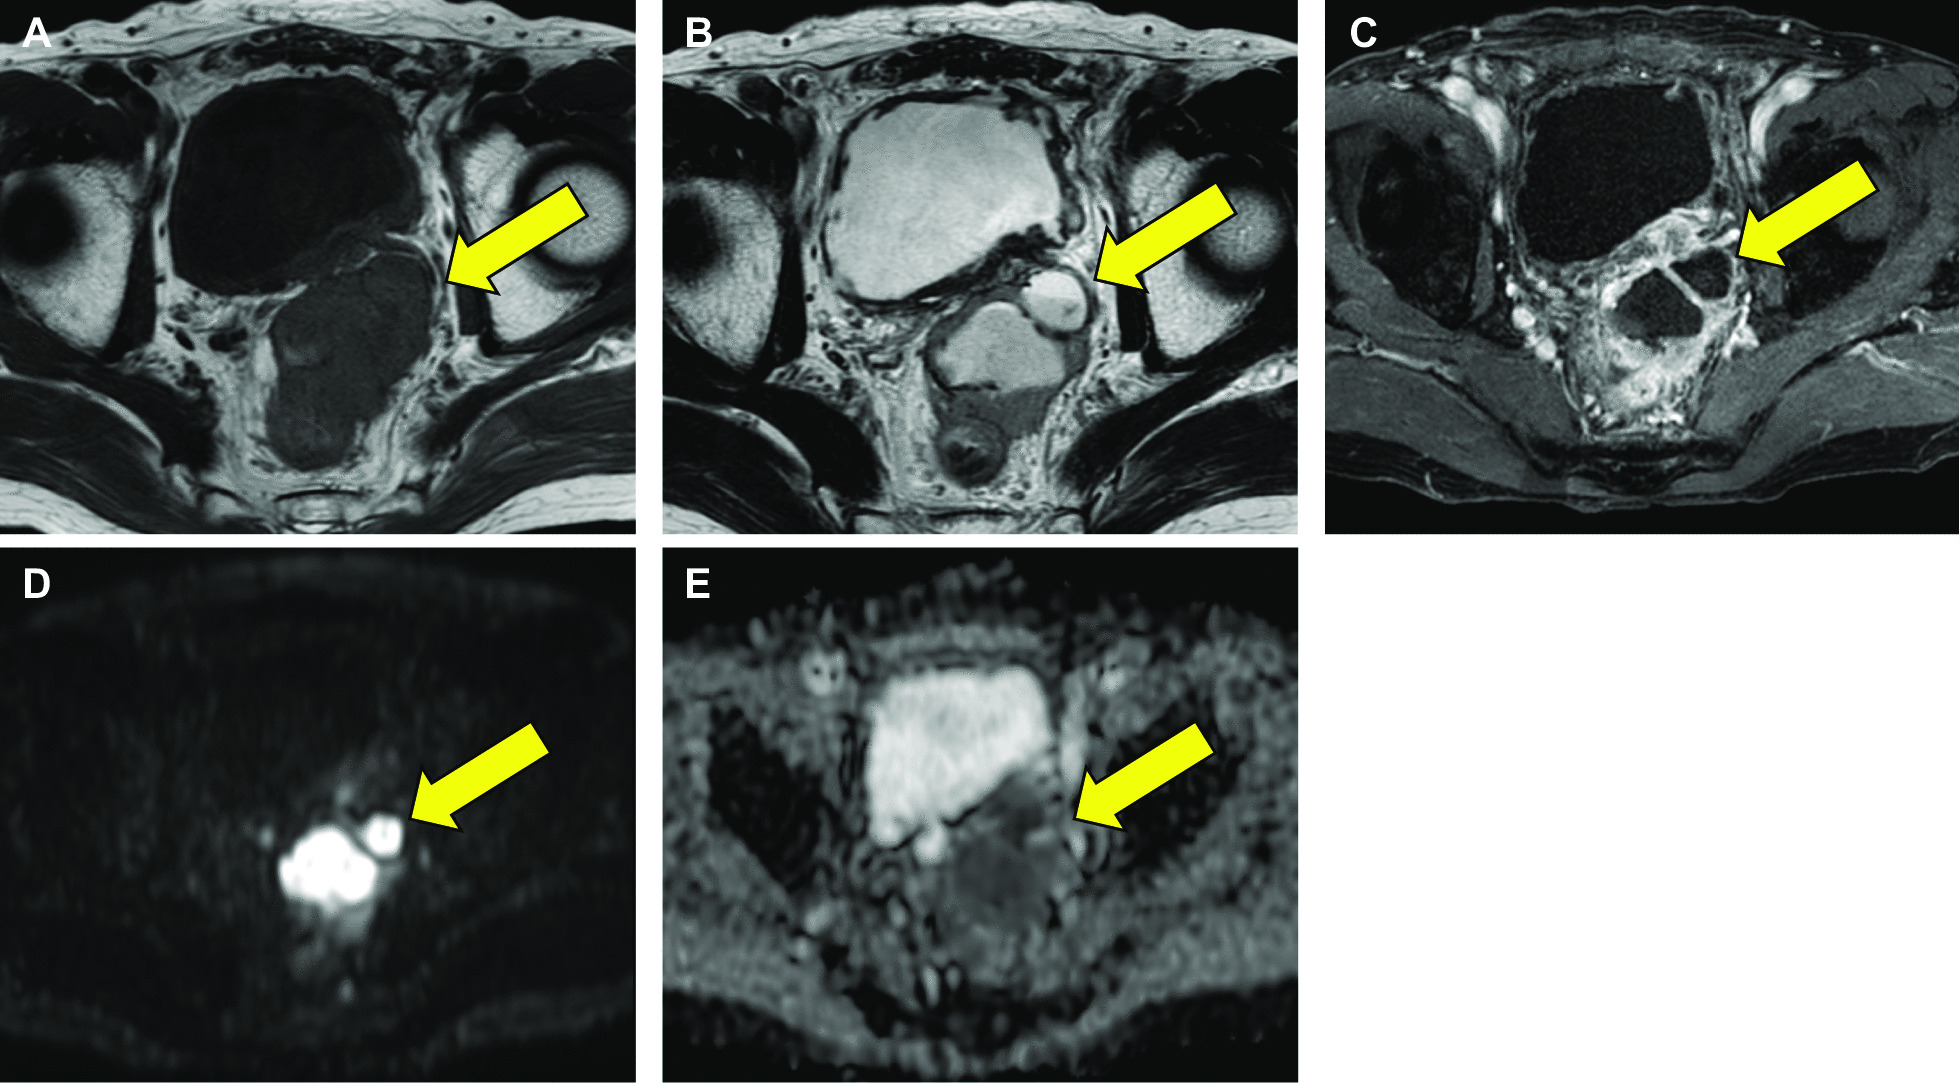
Contrast-enhanced MRI images: T1W (A), T2W (B), DCE (C), DWI (D), and apparent diffusion coefficient (ADC) map (E). The yellow arrow indicates the diverticular area. T1W, T1-weighted; T2W, T2-weighted; DCE, dynamic contrast-enhanced; DWI, diffusion-weighted image
